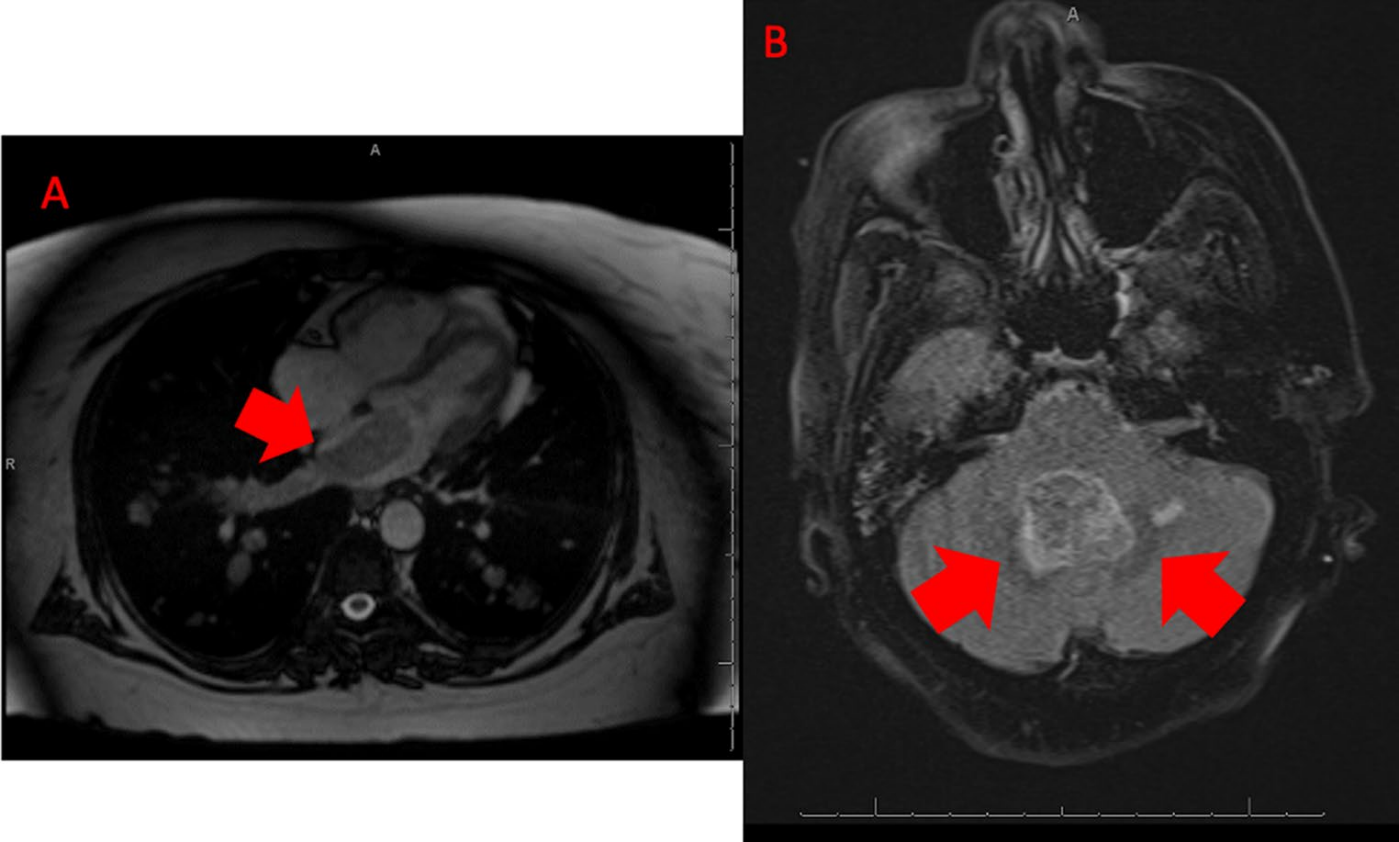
A) Cardiac MRI showing tumor indicated by red arrow arising from the pulmonary veins and into the left atrium. B) Brain MRI showing pontomedullary hemorrhage as indicated by red arrows
